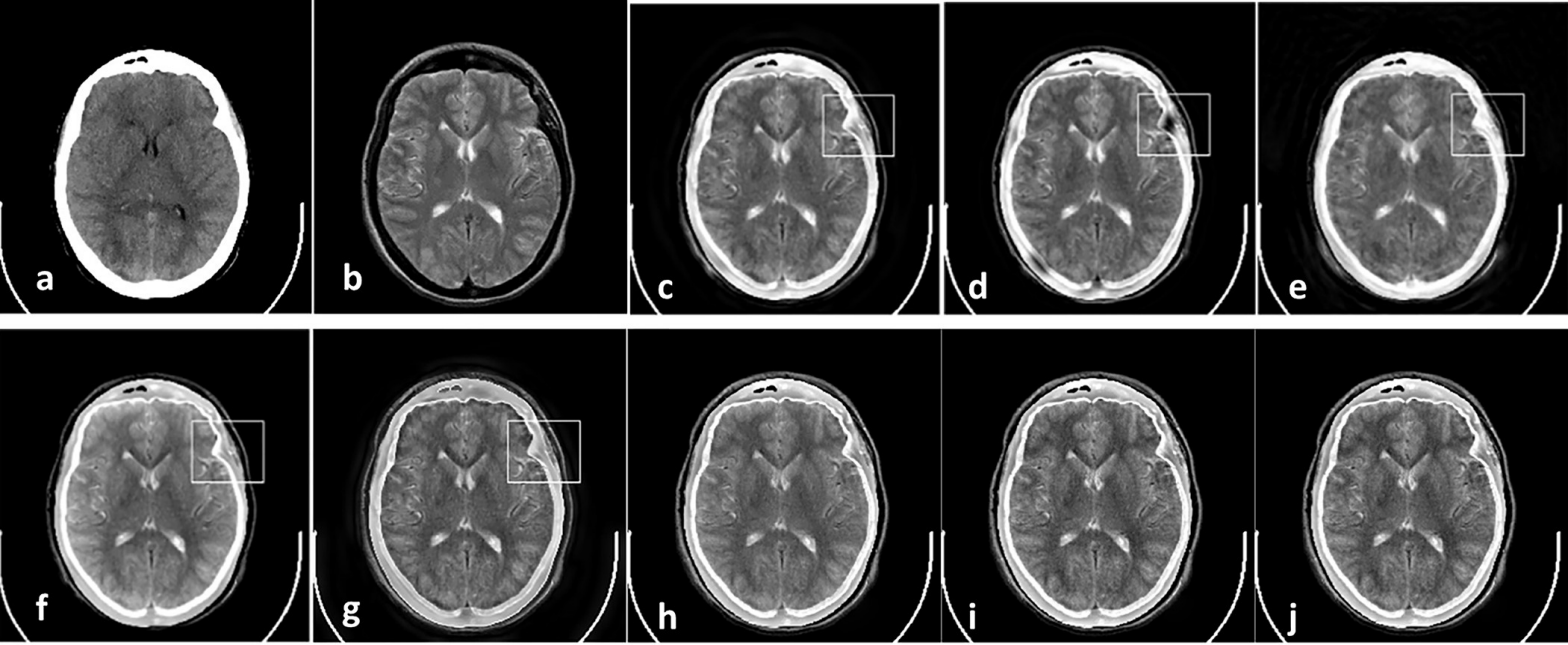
Experiment 8 [53]: Results of fusing CT and MR images of a brain. (a) CT image; (b) MR image; (c) NNSST [93]; (d) FMSAP [95]; (e) CST [97]; (f) ST-NSST [96]; (g) MFDF-NSST [53]; (h) the proposed method with PCA, (i) max, and (j) weighted averaging model, respectively.
